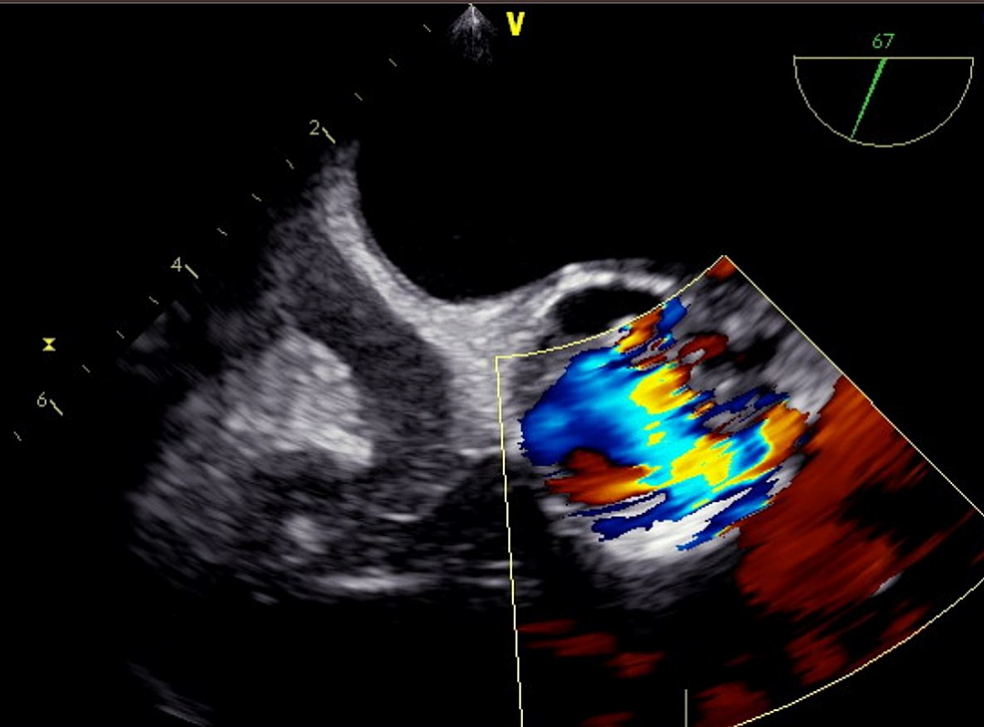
Transesophageal images at the mid-esophageal aortic valve short-axis view with the color Doppler box positioned over the aortic valve demonstrating continuous flow during systole and diastole, indicative of aortic insufficiency.
